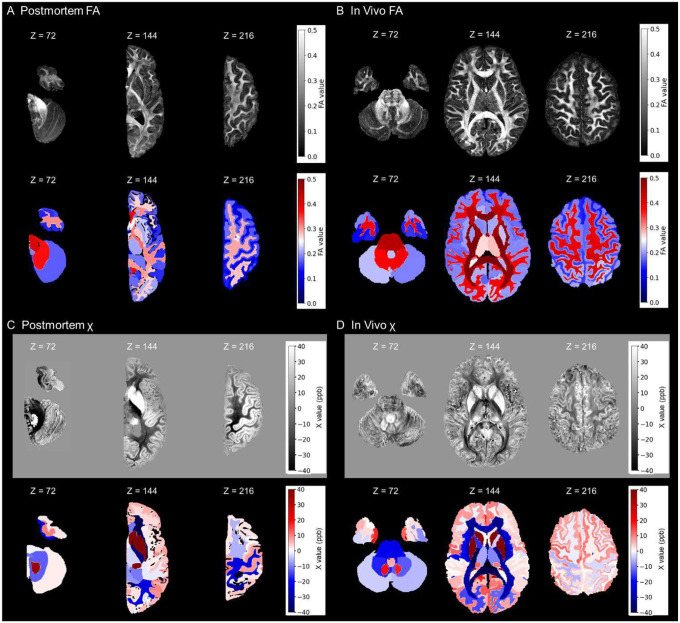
Atlas-based quantification of postmortem and in vivo FA and χ metrics Atlas-based analyses of FA and χ metrics constructed from postmortem and in vivo MRI data. (A) Postmortem FA and its atlas-based analysis of the postmortem human brain hemisphere. (B) In vivo FA and its atlas-based analysis of the healthy volunteer with matched submillimeter resolution. (C) Postmortem χ and its atlas-based analysis of the postmortem human brain hemisphere. (D) In vivo QSM and its atlas-based analysis of the healthy volunteer with matched submillimeter resolution. These quantitative maps are shown in the same anatomical orientation and location for visual comparison.
